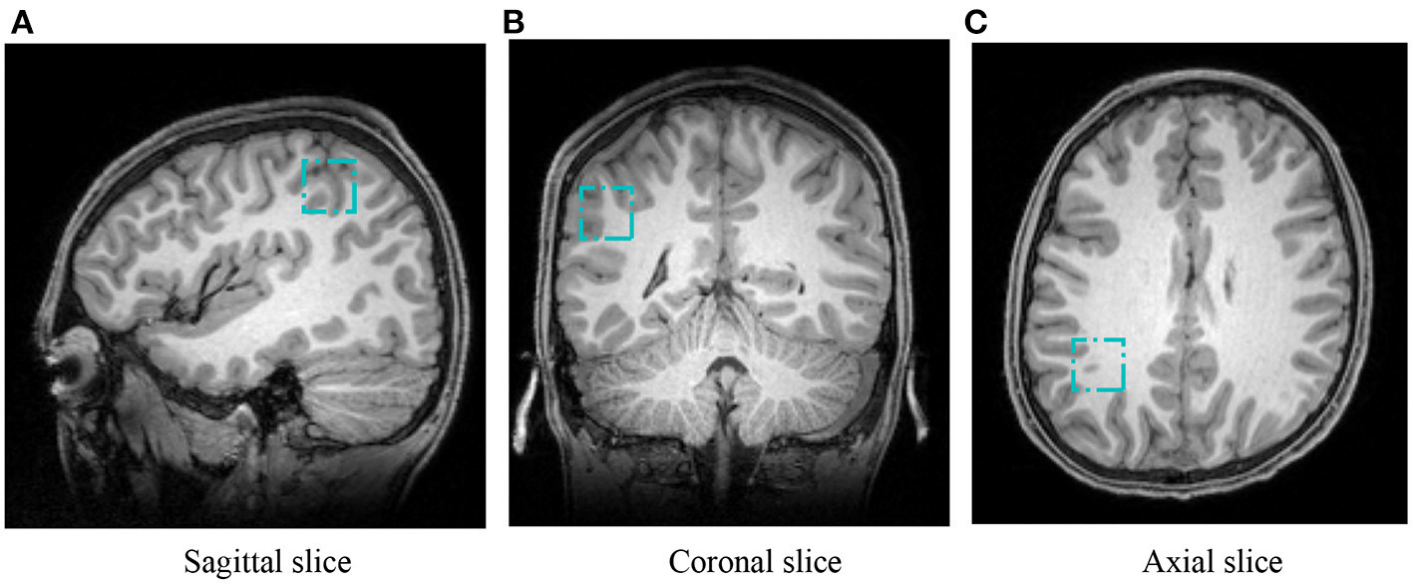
Positions of VOI in a representative T1-weighted MRI for IPS. Three cyan boxes show the IPS from sagittal, coronal, and axial views, respectively. (A) Sagittal slice, (B) coronal slice, and (C) axial slice.
